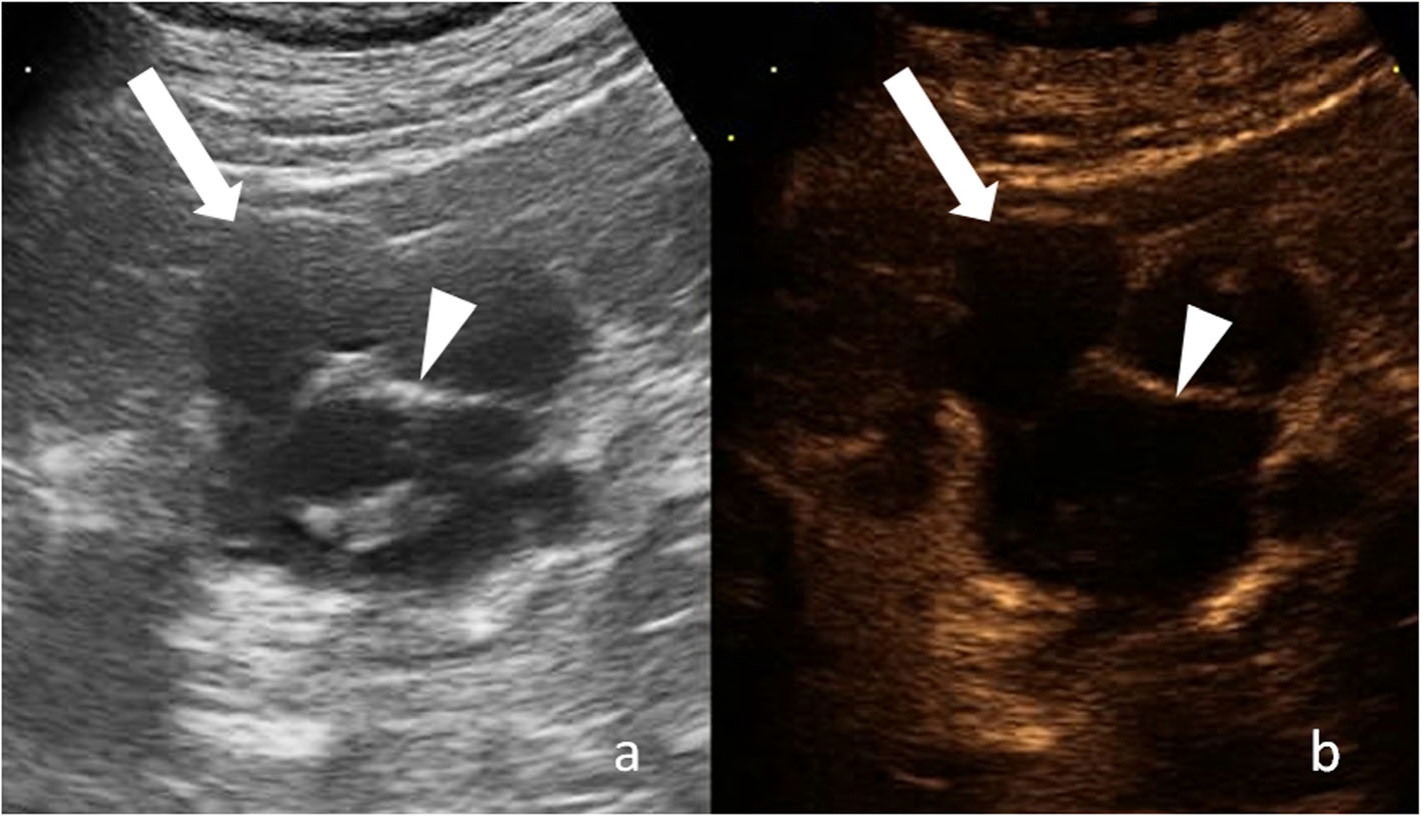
Cystic renal lesion in a 76-year-old-man. a Gray-scale ultrasound shows a cystic lesion (arrow) with a thin wall and thin septa (arrowhead), which contains fine calcifications. b Corresponding CEUS image shows enhancement of cyst wall and septa
